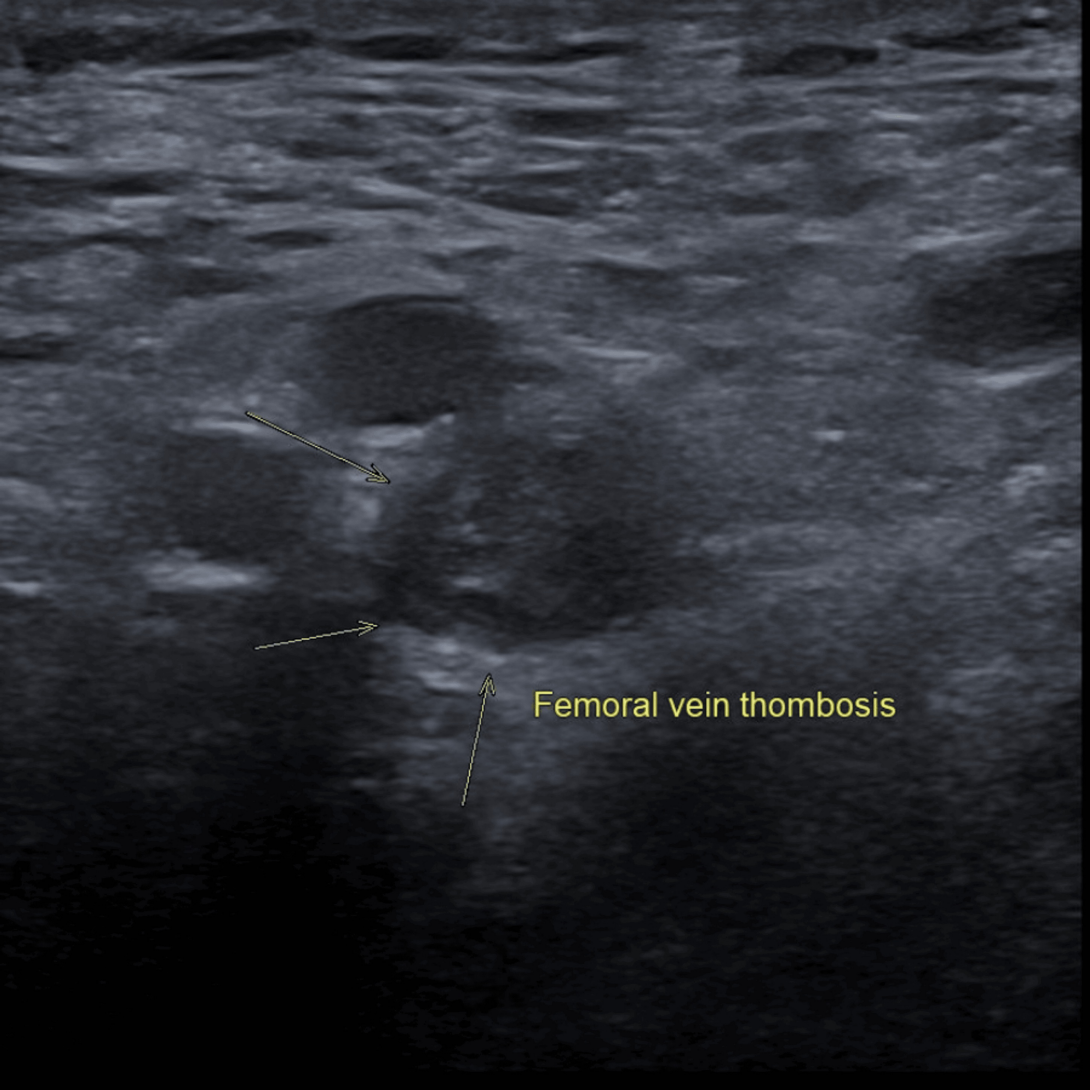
Sonographic Evidence of Femoral Vein Thrombosis
